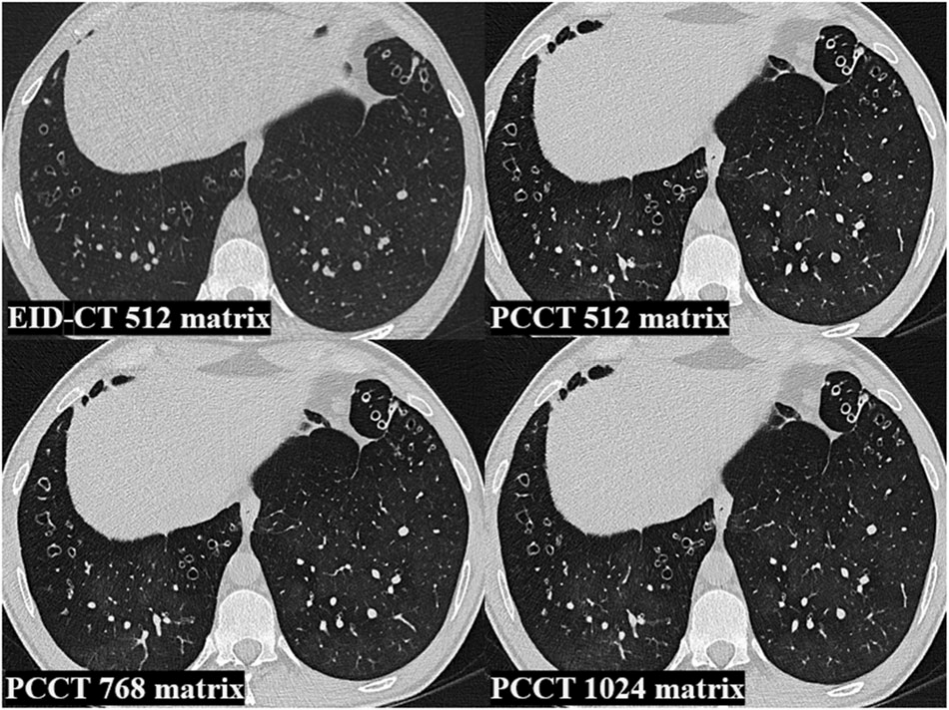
Examples of different reconstructions of chest CT of lower lobes with mosaic pattern. EID-CT, Energy-integrating detector CT; EID-CT 512, EID-CT 512 × 512 matrix; PCCT, Photon-counting CT; PCCT 512, PCCT 512 × 512 matrix; PCCT 768, PCCT 768 × 768 matrix; PCCT 1,024, PCCT 1,024 × 1,024 matrix
